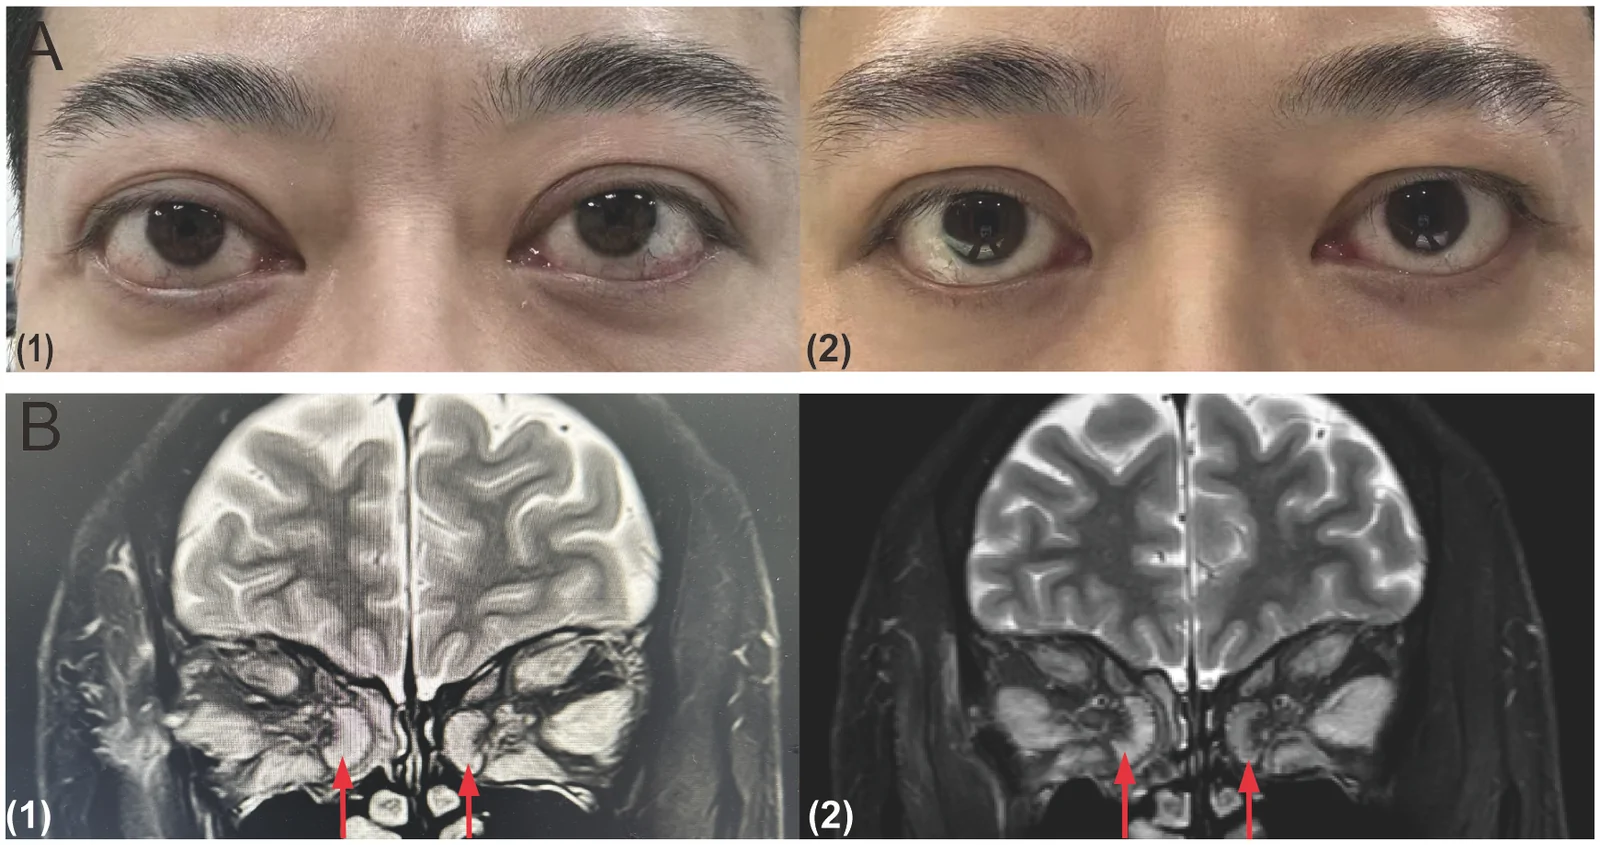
Clinical and radiological response to teprotumumab. (A) External ocular appearance before (A1) and after (A2) teprotumumab treatment. (B) Orbital MRI before (B1) and after (B2) teprotumumab treatment. Bilateral arrows indicate the medial rectus muscles and demonstrate reduced enlargement in both eyes after treatment.
